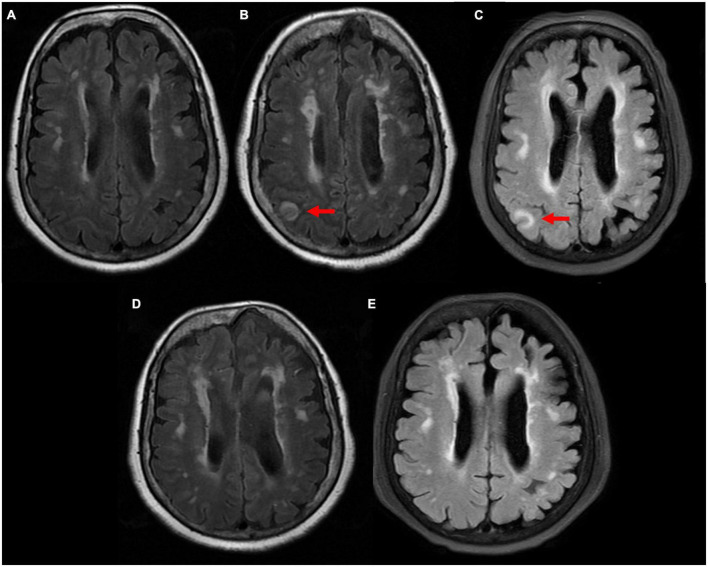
Comparison of magnetic resonance imaging (MRI) T2 flair sequence. (A) 4 years ago patient suffered from moderate headache and performed MRI scan in our clinic department. It showed multiple ischemic infarcts, white matter degeneration, brain atrophy, with no sign of abnormal signal. (B) 24 h after neurological symptoms, right parietal and occipital cortex swelling with abnormal signals was observed. (C) 72 h after neurological symptoms, right parietal and occipital cortex swelling with abnormal signals remained. (D) 12 days after neurological symptoms, abnormal signals disappeared. (E) 50 days after neurological symptoms, no sign of new abnormal signal.
